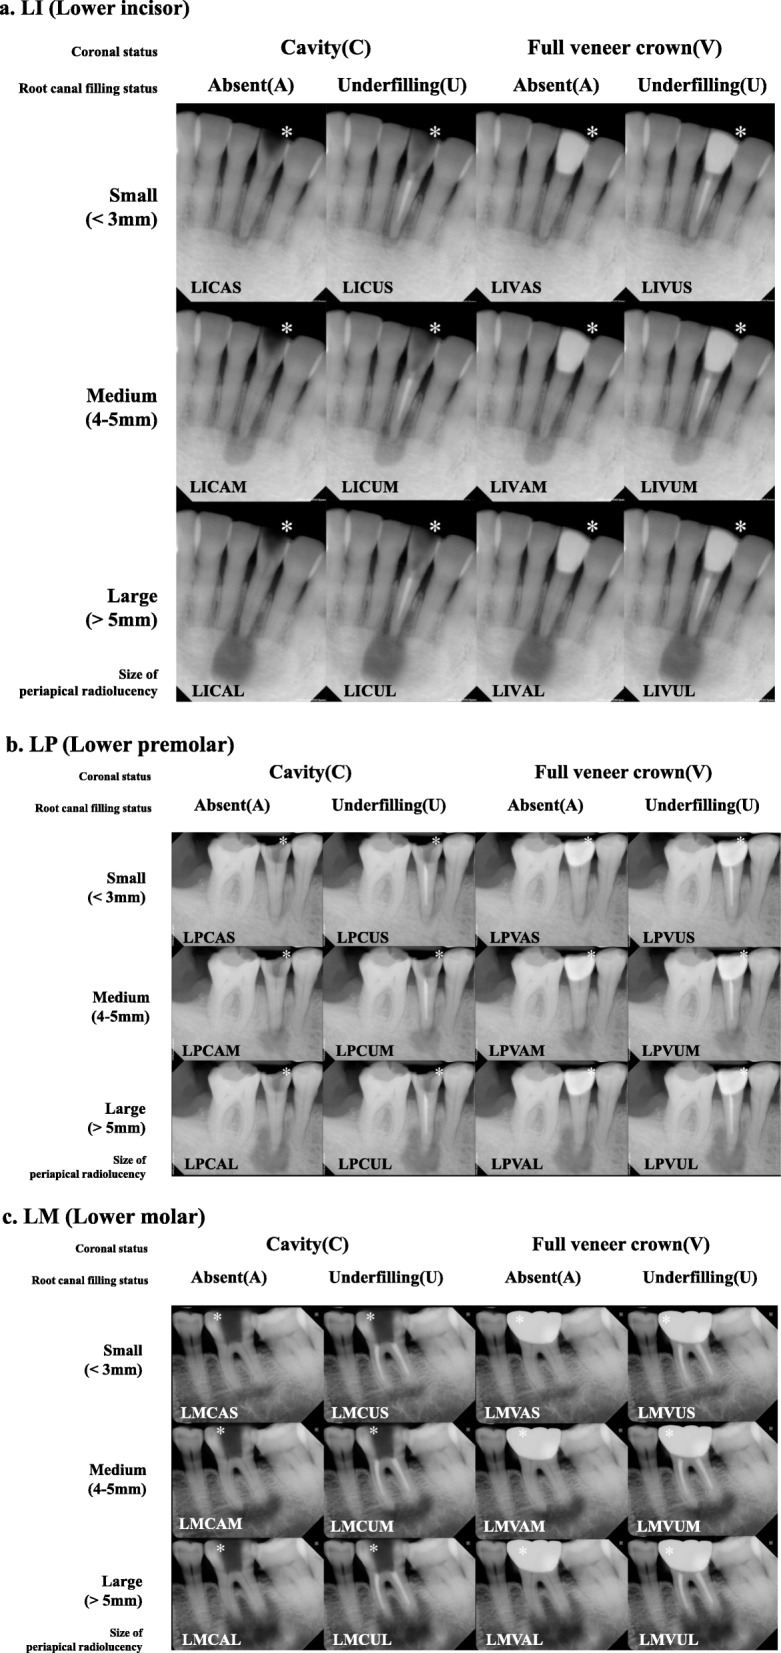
The 36 manipulated radiographs used in this survey. Each radiograph includes the abbreviations described in Table 1
